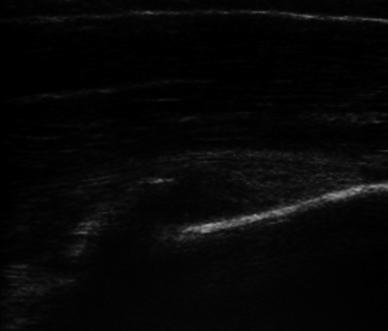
Ultrasound of the shoulder, showing a bony fragment within the muscle belly of the supraspinatus that is still attached to muscle fibers, and an interrupted cortical line of the scapular spine
